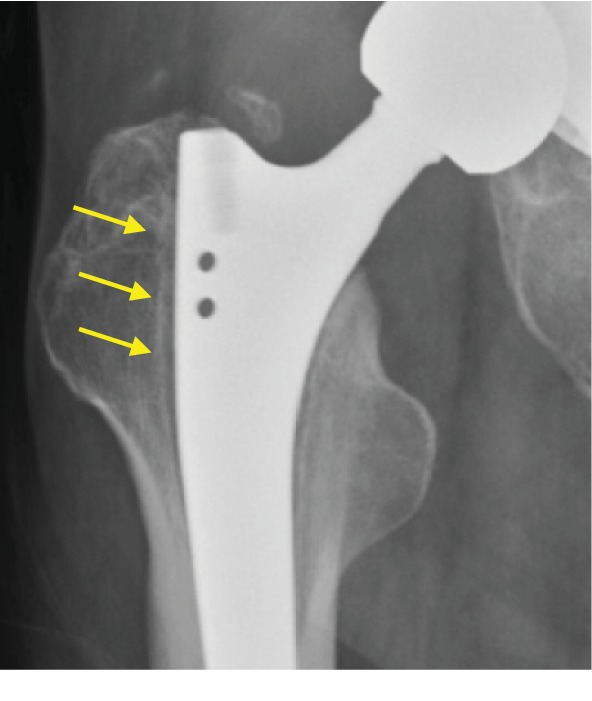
A 62-year-old female treated with non HA SL-PLUS MIA stem under the diagnosis of right hip osteoarthritis. On radiography, there was radiolucent line around proximal area accompanied by reactive line (yellow arrows).
